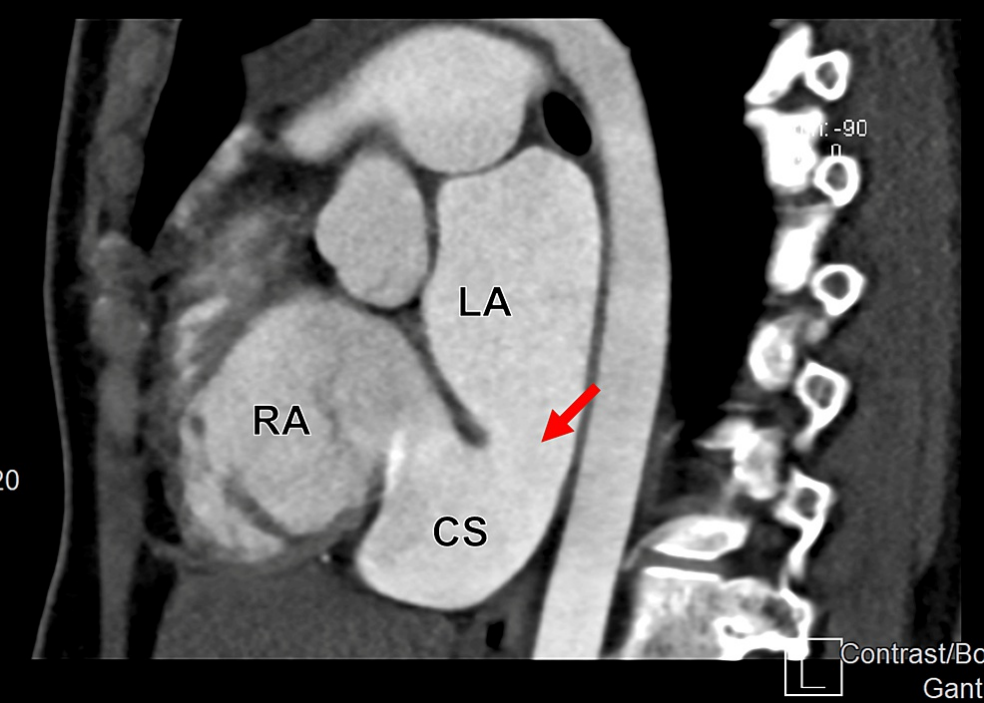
Cardiac computed tomography angiography (CCTA) in a sagittal display showing a communication between the RA and LA, representing an unroofed CS (arrow). RA: Right atrium; LA: Left atrium; CS: Coronary sinus.
